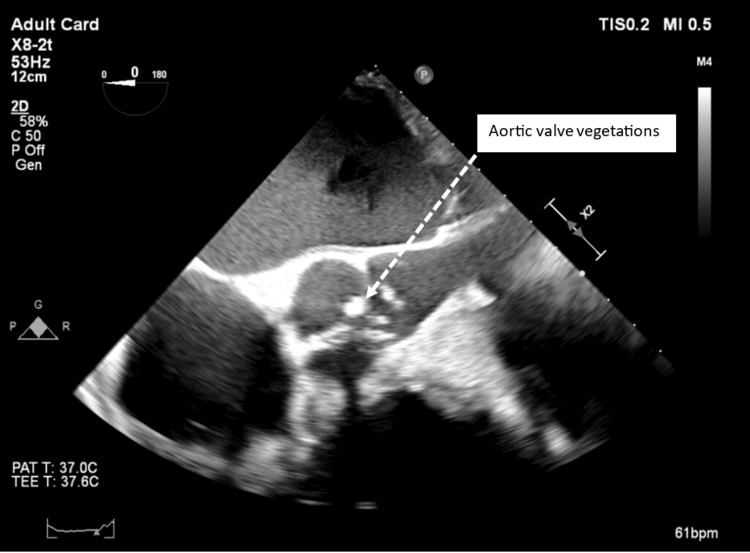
Transesophageal echo obtained on day 21 of hospitalization demonstrating multiple mobile vegetations on the aortic valve.
